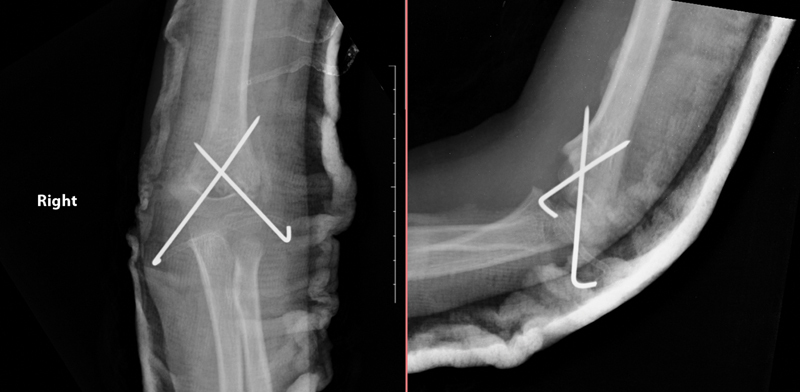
Anteroposterior and lateral radiographs of the same patient in fig. 3 three weeks after fixation, demonstrating reduction loss.
